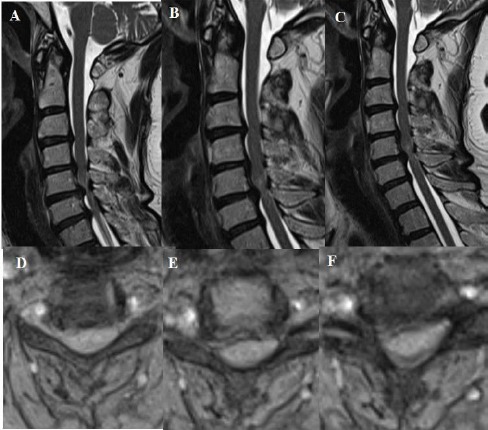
A, B, C, D, E, F: T2 weighted sagittal and axial MRI showing Compression to the medulla spinalis at four consecutive levels which are from cervical 3 and cervical 4 to cervical 6 and cervical 7. Osteophyte formation and posterior longitudinal ligament ossification lead to this compression on medulla spinalis. Besides, cervical lordosis angle was measured 20.9 degrees on lateral X-ray at the preoperative period*.
